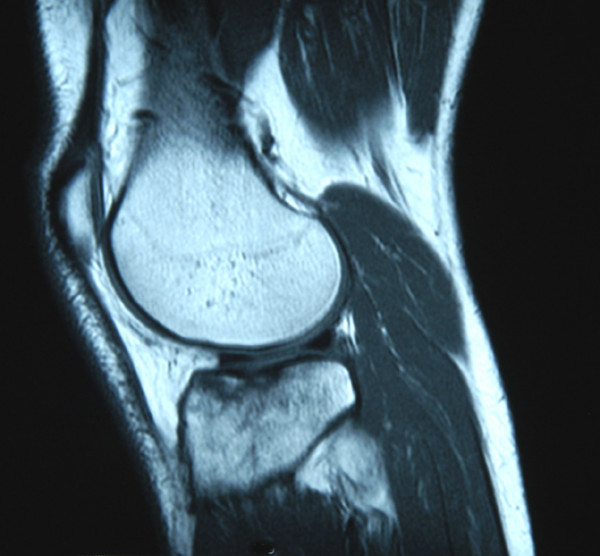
Sagittal T1-weighted sequence MRI demonstrating extensive bone marrow oedema within the lateral tibial plateau extending to the articular cartilage.
